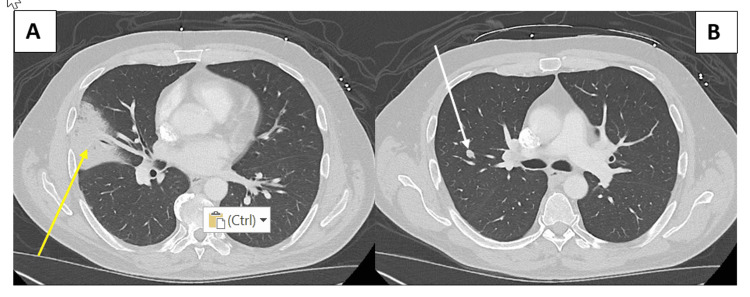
Axial computed tomography images of the chest demonstrating a consolidation in the right mid-lung zone (A, yellow arrow) and a small nodule in the upper right lung (B, white arrow)
